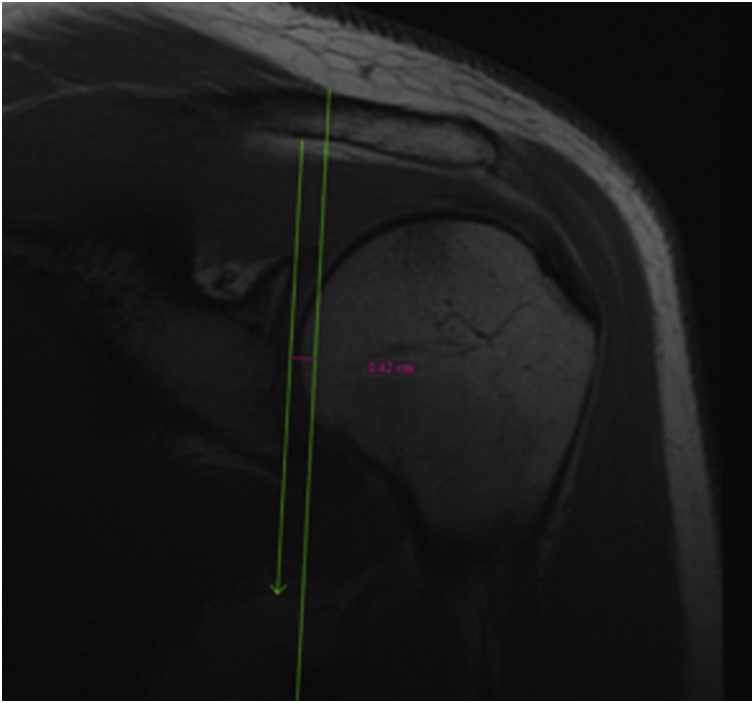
Glenoid depth as seen on the coronal view on shoulder MRI.
